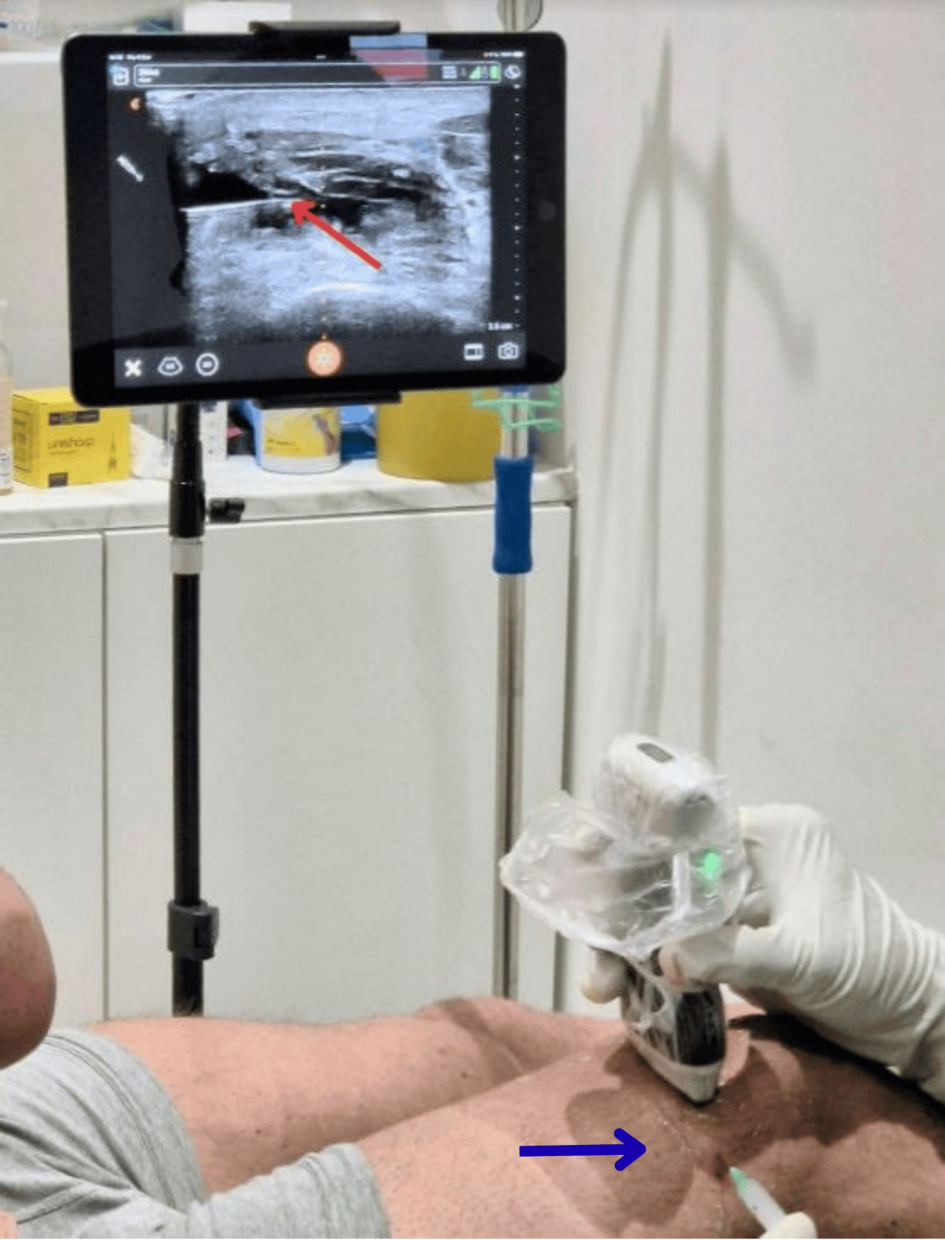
Suprapatellar recess technique (lateral-to-medial in-plane approach) for medication delivery by injection under ultrasound guidance (blue arrow) in the knee joint The needle well can be seen on the monitor screen as it enters the suprapatellar bursa (red arrow).
